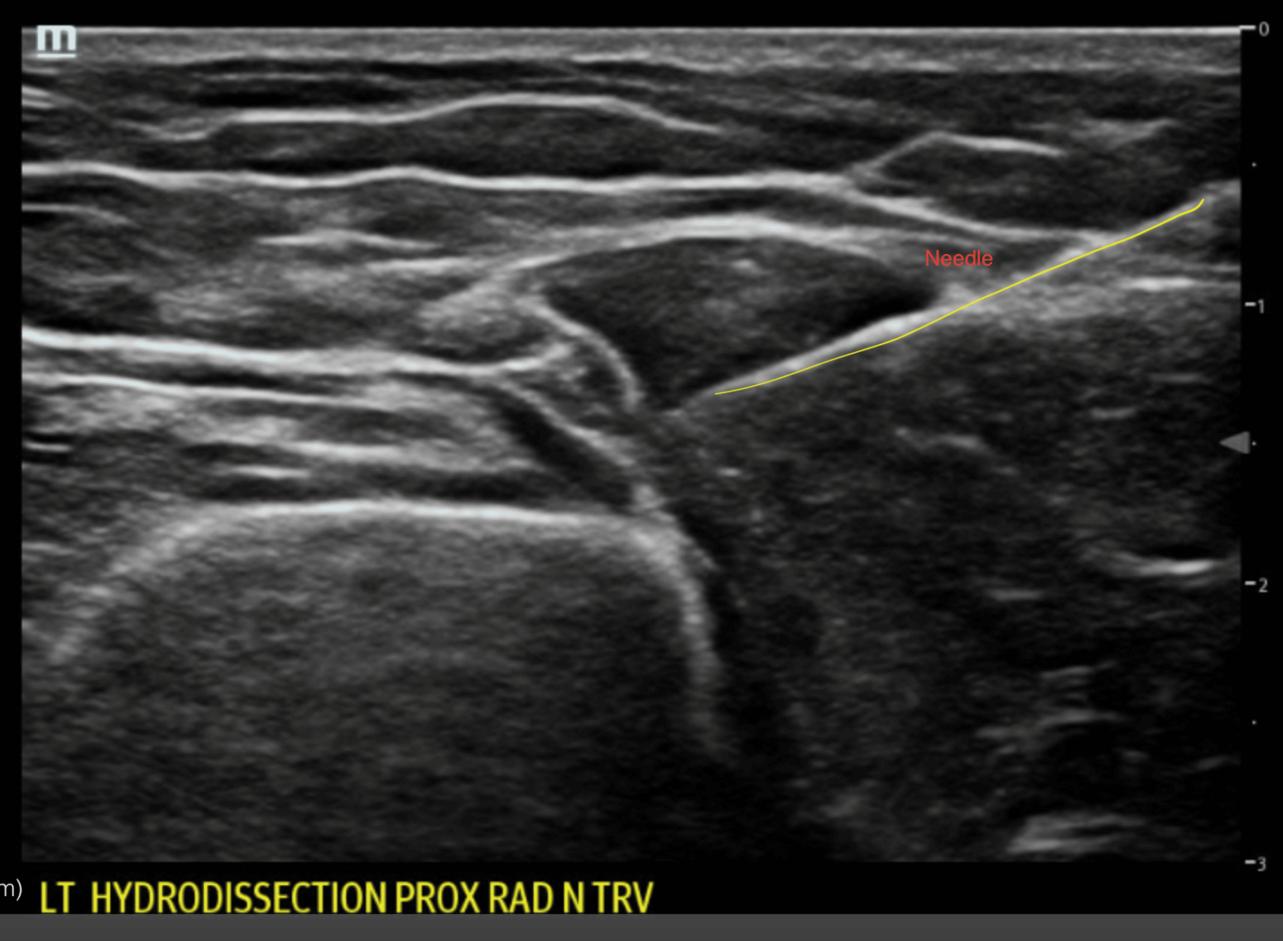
Ultrasound showing hydrodissection with additional fluid adjacent to the radial nerve
